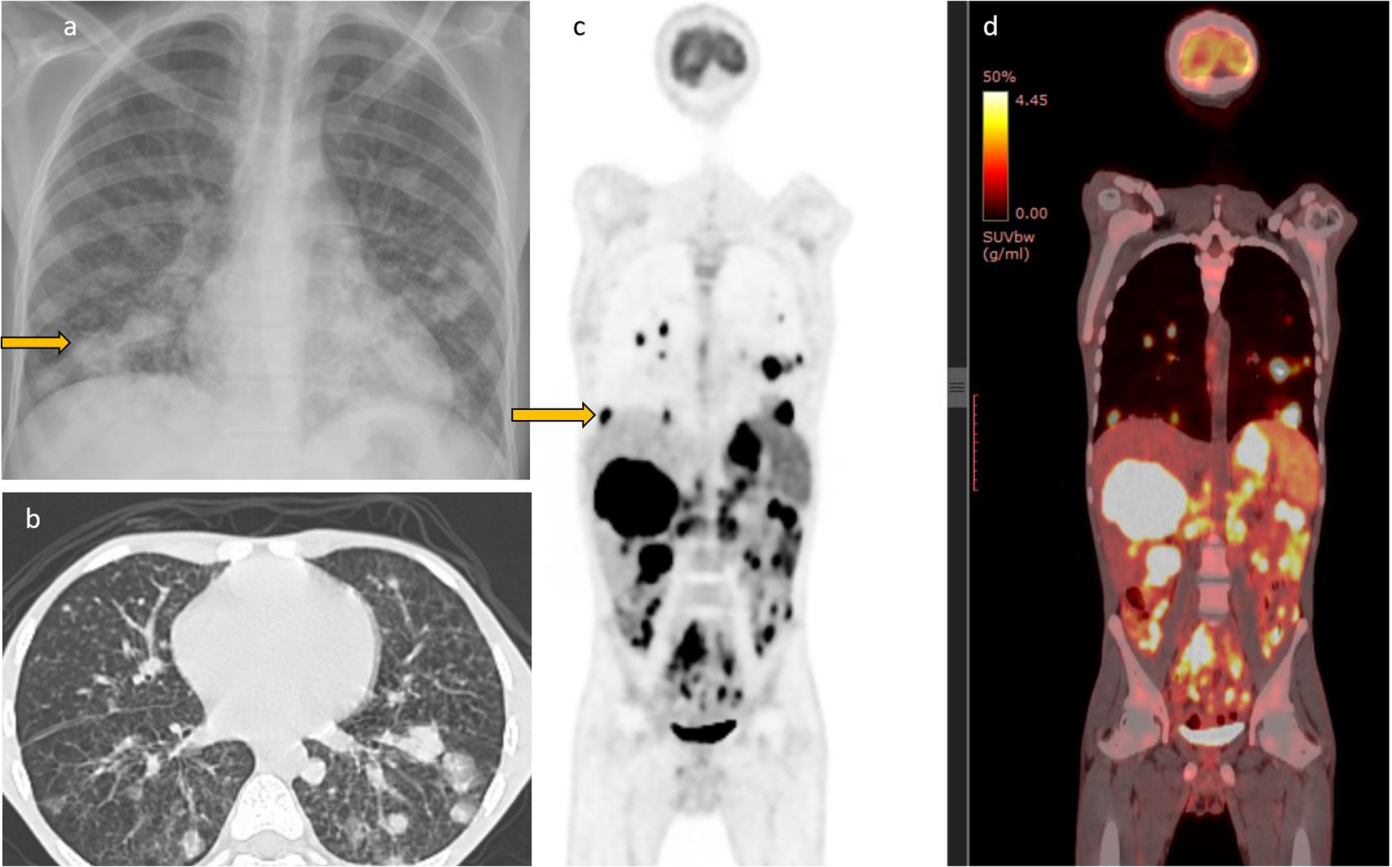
Chest radiograph of a 19-year-old HIV-positive patient with low CD4 count. He presented with cough and dyspnea and was initially being evaluated for possible atypical pneumonia. The chest radiograph showed multiple focal opacities predominantly in the lower lung zones. On CT, there were lung parenchymal nodules/masses of variable sizes which were also intensely FDG avid on PET CT. There was extensive lymphoma involvement elsewhere including in the liver, spleen (splenic activity higher than liver background), and multiple nodal sites above and below the diaphragm. Biopsy of the liver lesion was consistent with DLBCL
